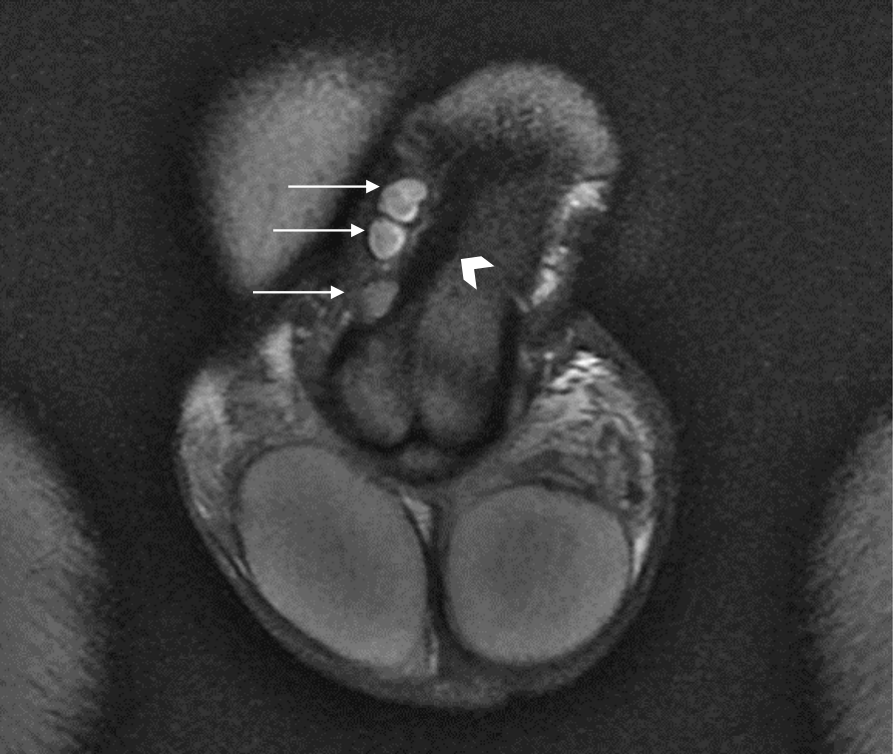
Coronal T2-weighted magnetic resonance image (MRI) of penile nodules. Coronal T2-weighted MR image shows three well-defined T2W hyperintense nodules (arrows) within the penis. These are superficial to the tunica albuginea (arrowhead)
